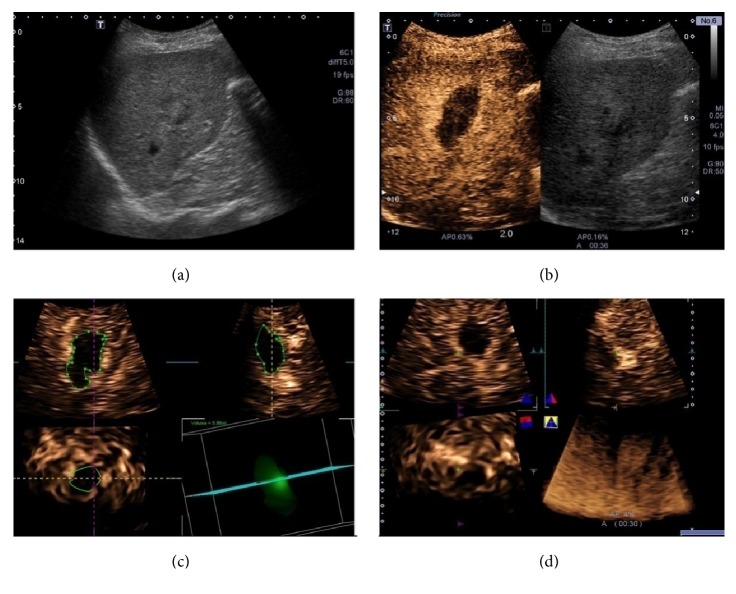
Complete response after RFA. B mode ultrasound image in a 65-year-old man showed a 25 mm sized inhomogeneous hypoechoic lesion in the right hepatic lobe (a). 2D-CEUS showed the nonenhanced necrotic area was larger than the target lesion (b). The contour of the ablative zone was depicted manually and its volume was 5.88ml (c). “Inversion” mode showed the nonenhanced area on 3D-CEUS image (d).
